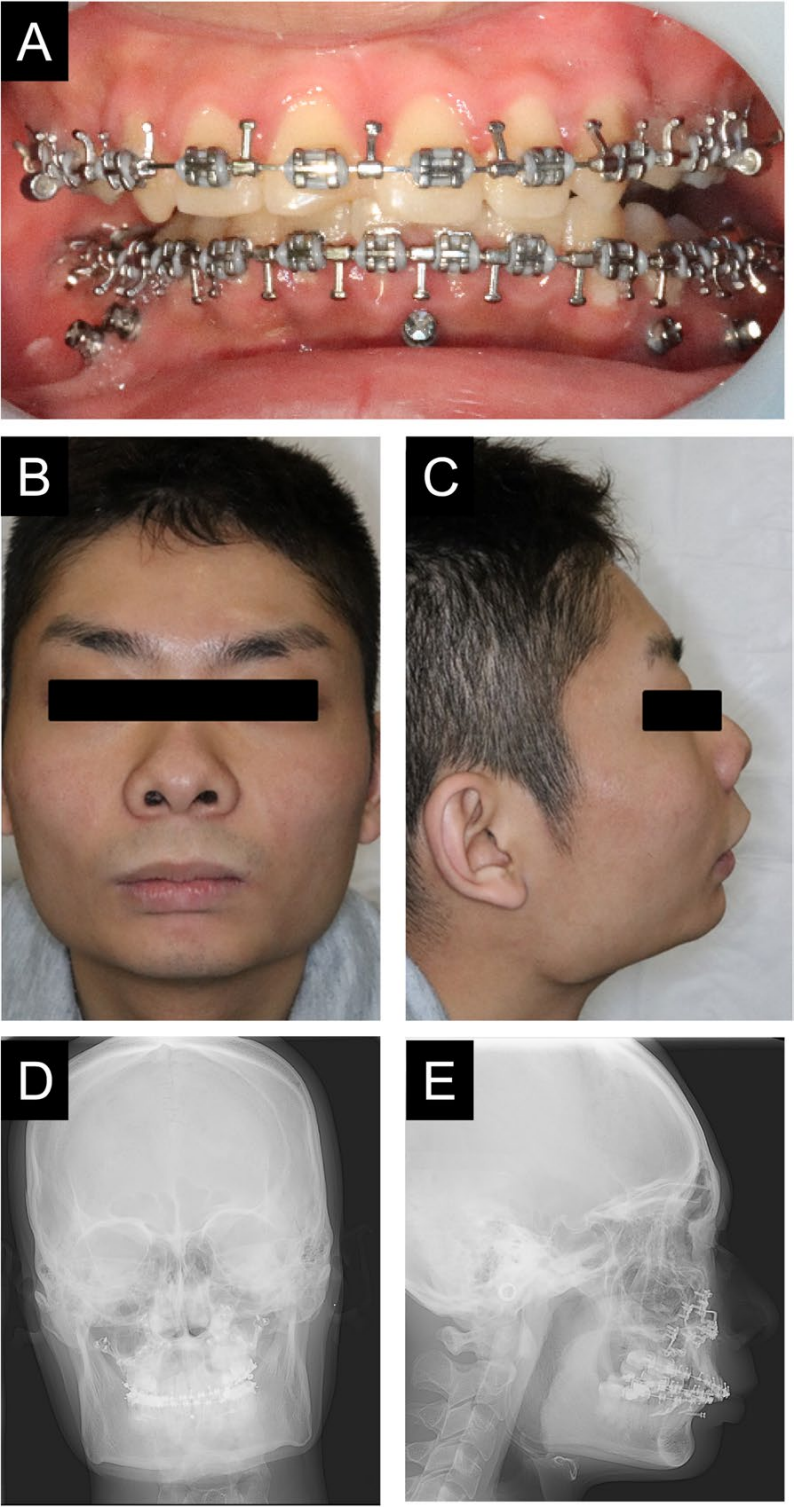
During treatment photographs and radiographs. Intraoral photographs before mandibular osteotomy (A). Frontal view (B) and lateral view (C) on facial photographs before mandibular osteotomy. Frontal cephalogram (D) and lateral cephalogram (E) showing radiographic findings before mandibular osteotomy
